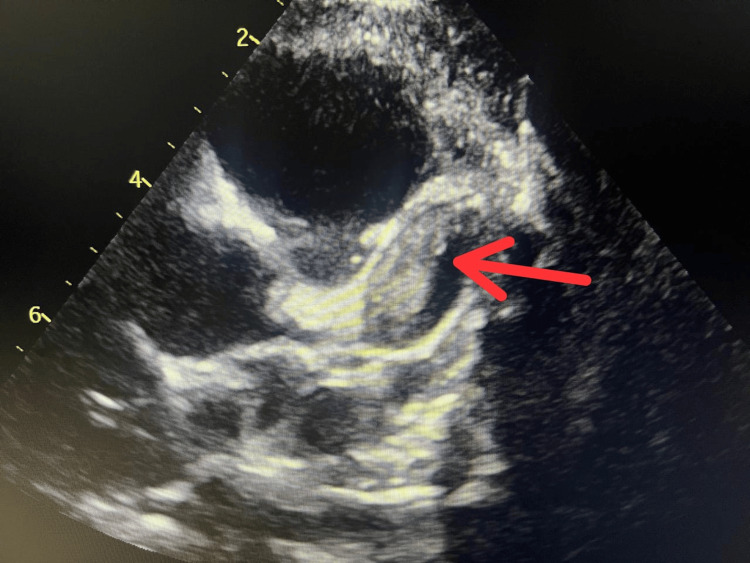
Echocardiographic image of the patient This echocardiographic image reveals a dilated left anterior descending (LAD) artery with a distinct echogenic structure within the lumen, consistent with thrombus.
